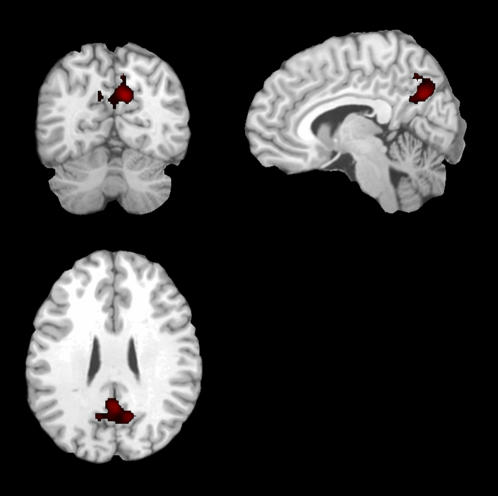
Posteromedical cortex (PMC), denoted in red and overlaid on a canonical T1-weighted brain template image in three orthogonal views, was used as an apriori functional region of interest. In this region parameter estimates for activation magnitude showed a lesser-to-greater activation from Control, to MCI, to AD subjects.
